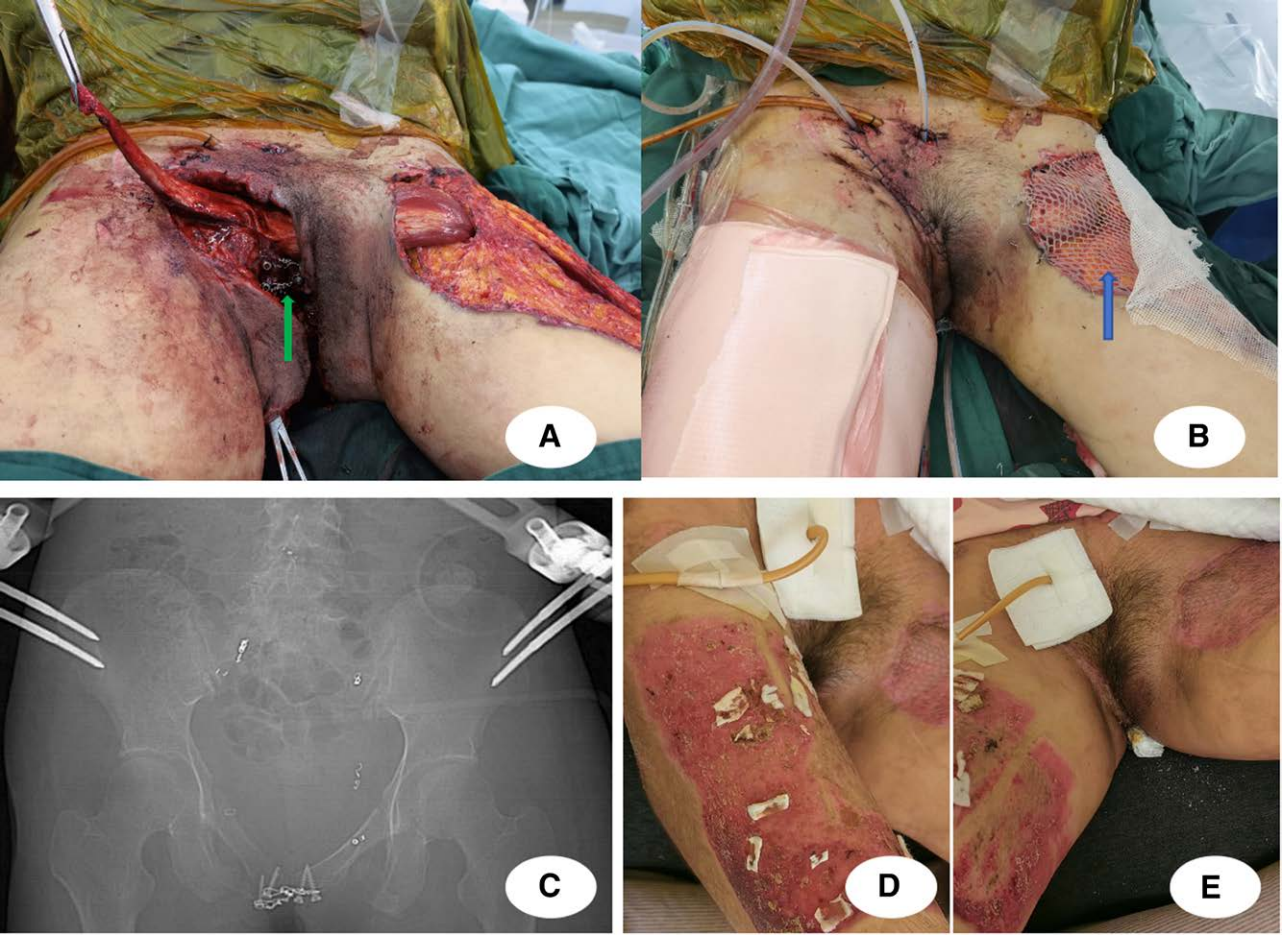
(A) Postoperative radiographs demonstrating bone stabilization. (B) Reconstruction and internal fixation of the pubic symphysis, with transposition of the left rectus femoris muscle flap (red arrow). (C) Wound closure achieved through split-thickness skin grafting (blue arrow). (D) and (E) Demonstrate successful wound healing.
